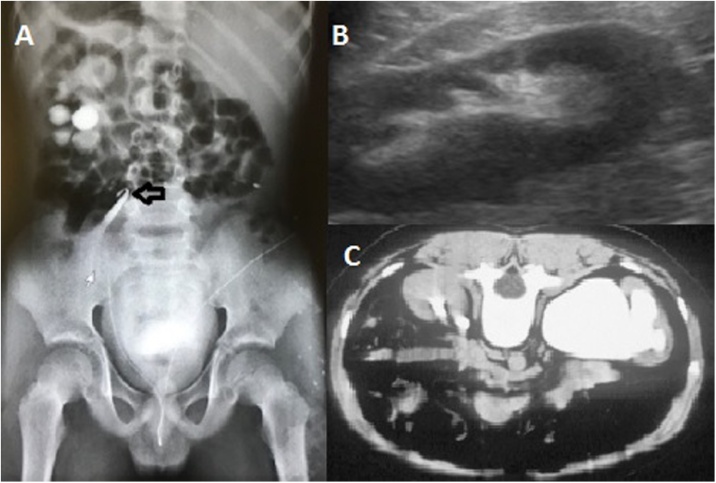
(A) Ultrasonographic showing a moderate left hydronephrosis with thin renal cortex; (B) Retrograde ureteropyelography revealing ureteral papilloma, left upper-ureteral filling defect of the ureter; (C) Abdominal computed tomography scan confirmed a filling defect lesion with smooth margin in the proximal left ureter and left hydronephrosis.
